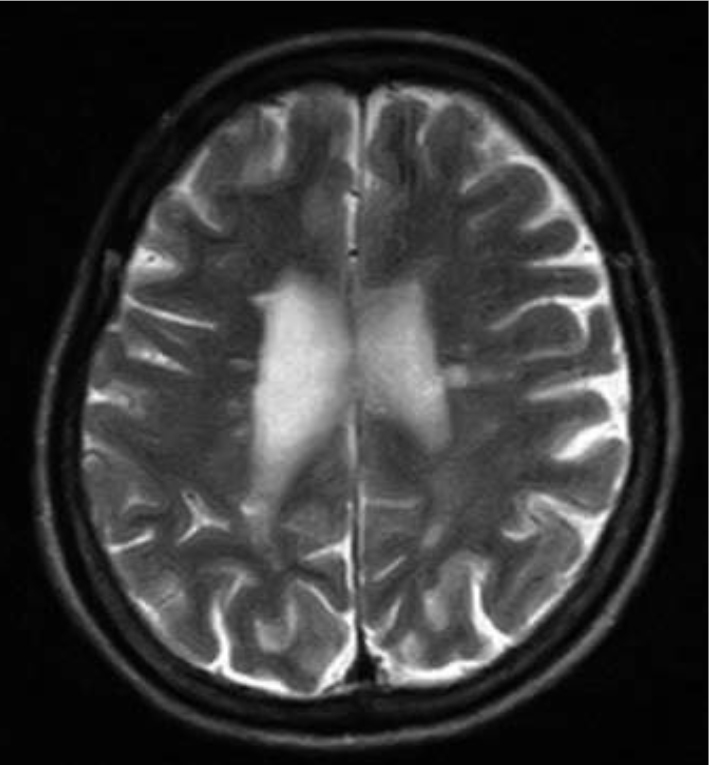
Brain MRI(T2-weighted)revealed multiple hyperintense white matter lesions adjacent to the bilateral ventricles at 44 years of age.
